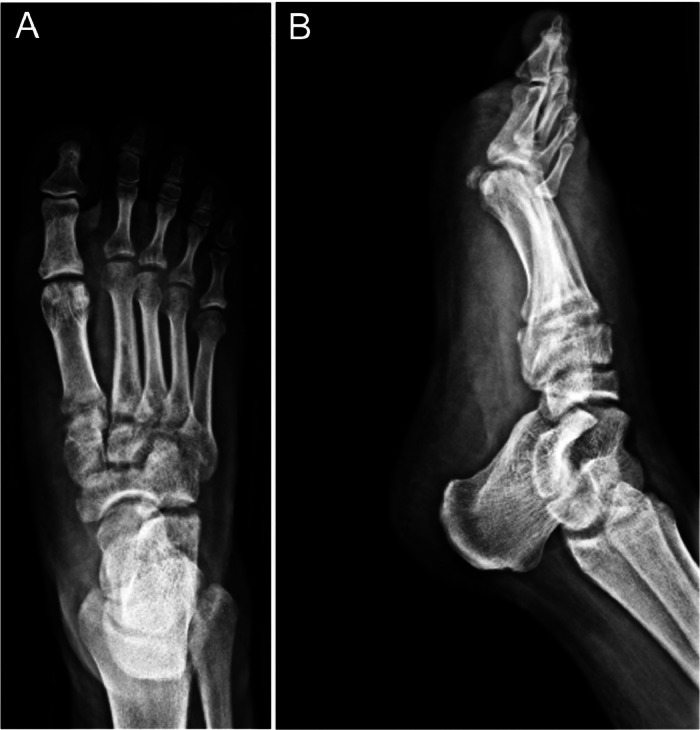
Follow-up plain radiographs of the right foot taken 6 weeks postoperation. (A) Anteroposterior (AP) view, (B) Lateral view. Pins were removed 6 weeks after surgery, and weight-bearing activities were initiated. The medial cuneiform and metatarsals remain in normal alignment, with no abnormalities observed.
